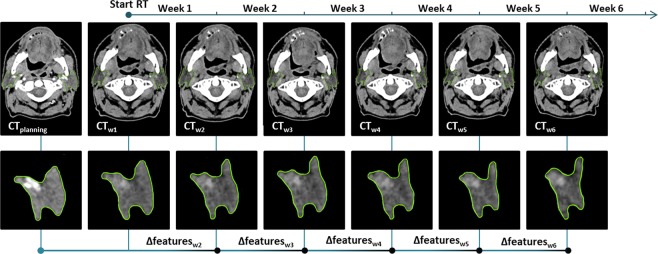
The difference between features extracted from the weekly CTs (CT_w._) and either the planning (geometric features) or week 1 CT (intensity and texture features) resulted in ∆features per week. Generally, scans were taken at the start of consecutive radiation week.
